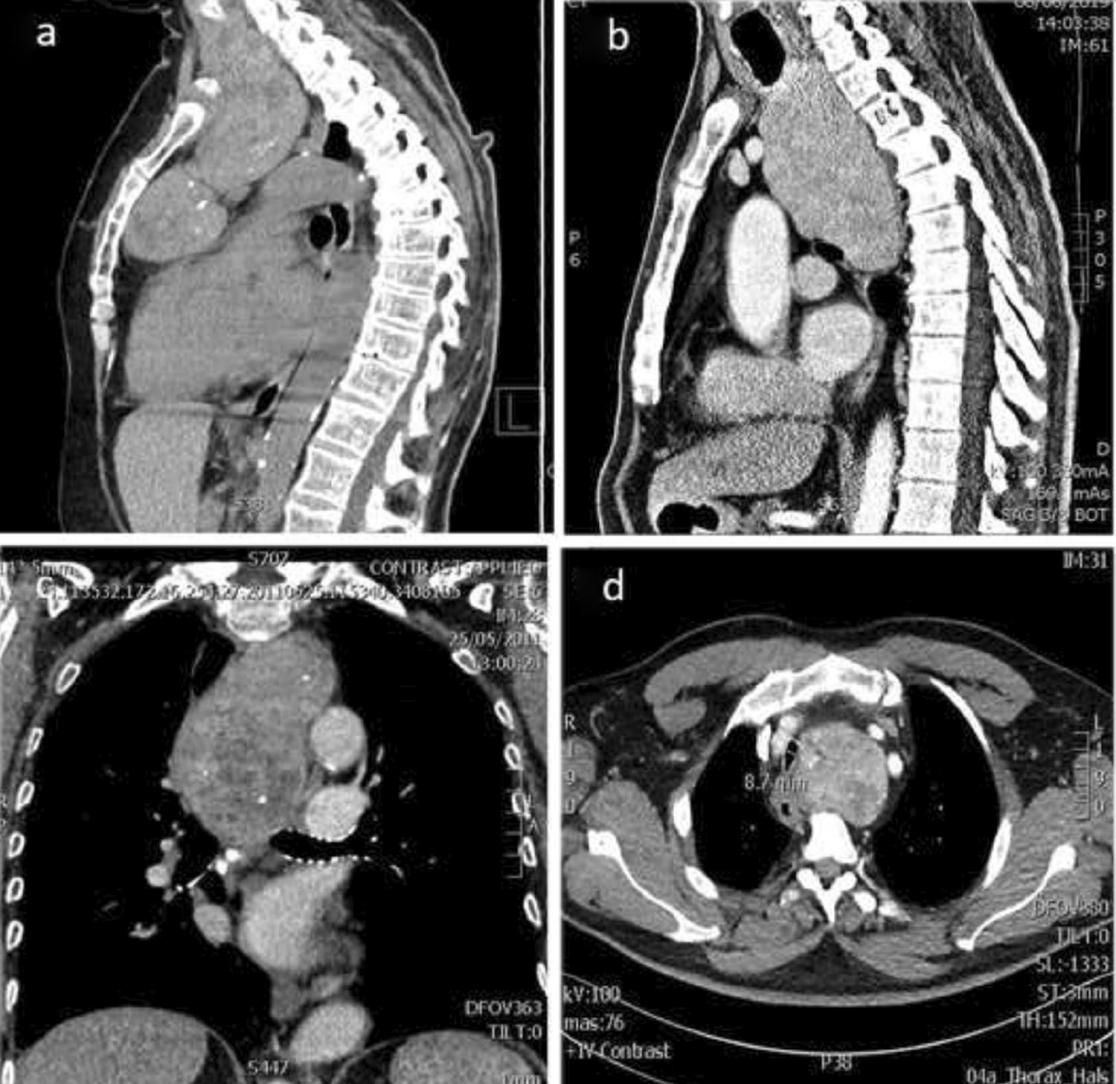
Preoperative Computed Tomography: intrathoracic goiter (a) in anterior mediastinum, (b) in posterior mediastinum, (c) extending below the carina (d) causing trachea deviation and narrowing
